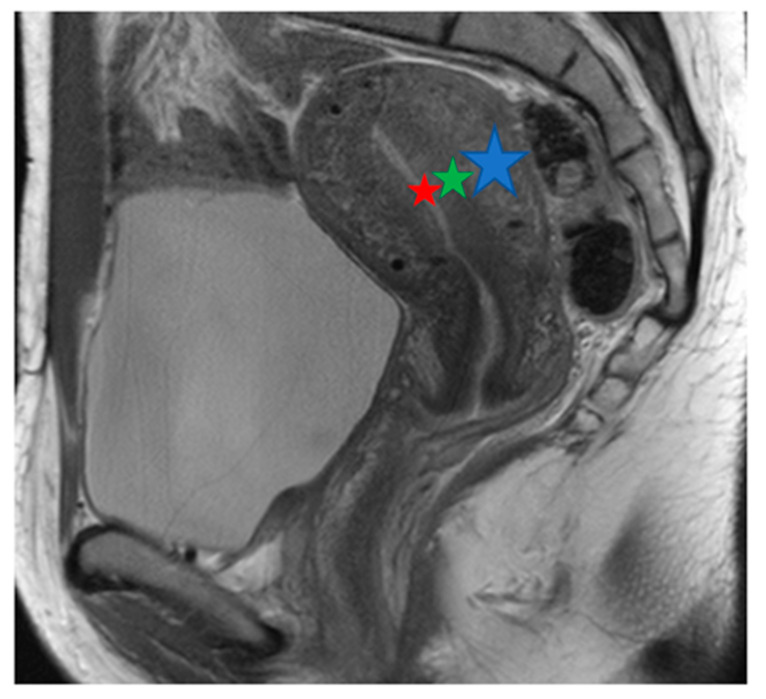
Normal uterus: T2w sagittal image. MRI displays the zonal anatomy of the uterus. The endometrium has a high T2 signal (red star); the inner myometrium has a low T2 signal, known as the junctional zone (green star). The outer myometrium has an intermediate signal on T2 (blue star).
